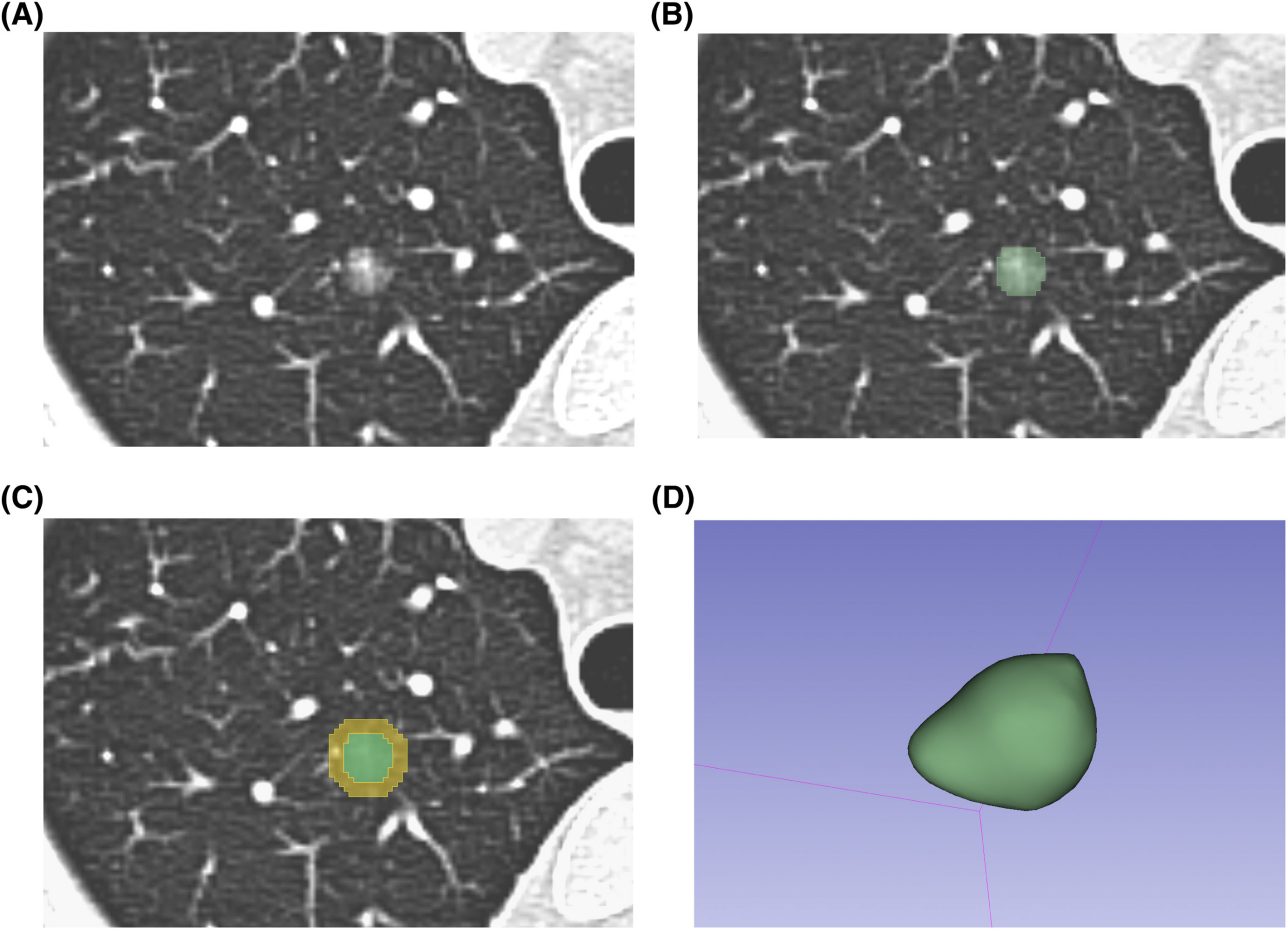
Segmentation process of the intra‐nodular and peri‐nodular regions in 3D slicer. (A) A ground‐glass opacity nodule (GGO) on the 1.5 mm CT slice. (B) Segmentation of the intra‐nodular region (marked green). (C) Peri‐nodular region was acquired by extending the intra‐nodular region by 5 mm from its boarder in 3D using function “Hollow” (marked yellow). (D) The 3D model of the delineated GGO
